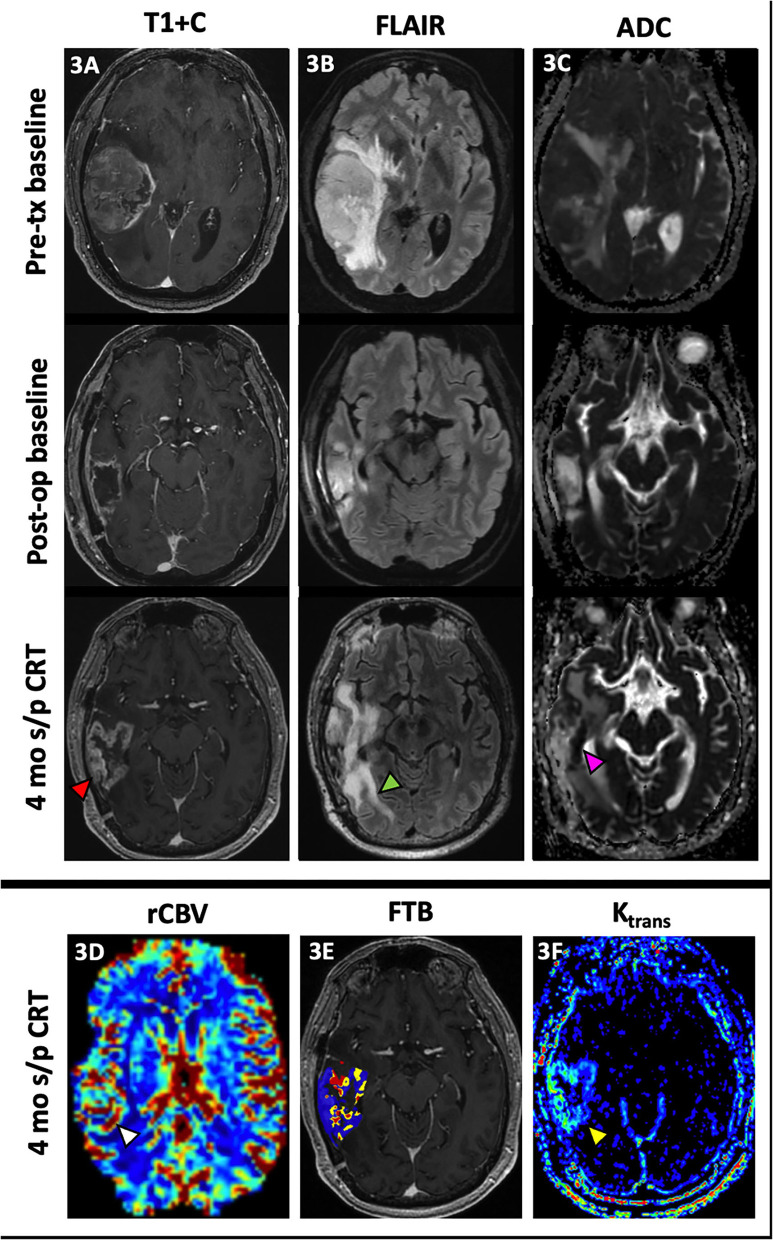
Admixture of treatment effect and tumor. A 68 year-old male with GBM (IDH wt, MGMT+) initially presented with a large enhancing right temporal lobe mass [(A), top row] with extensive perilesional FLAIR hyperintensity [(B), top row] and diffusion restriction [(C), top row]. After resection, there is mild peripheral enhancement of the resection cavity [(A), middle row] with decreased perilesional FLAIR hyperintensity [(B), middle row] and no significant diffusion restriction [(C), middle row]. At 4 mo s/p CRT, new peripheral nodular enhancement of the resection cavity [(A), bottom row, red arrowhead] is noted with interval increase in perilesional FLAIR hyperintensity [(B), bottom row, green arrowhead] and increased diffusion restriction most pronounced at the medial aspect of the resection cavity [(C), bottom row, pink arrowhead]. There is also associated increased rCBV [(D), white arrowhead], a mix of low and high fractional tumor burden (blue and red, respectively) (E) and vascular permeability [(F), yellow arrowhead]. This lesion was resected with pathology demonstrating predominantly radiation necrosis (Ki-67 of 5%) but with some neoplastic cells. The heterogeneous nature of this treated lesion is supported by the mix of low and high fractional tumor burden seen on the FTB map. ADC, apparent diffusion coefficient; CRT, chemoradiotherapy; FTB, fractional tumor burden; GBM, glioblastoma; IDH, isocitrate dehydrogenase; K^trans^, volume transfer coefficient; MGMT, O-6-methylguanine-DNA methyltransferase; mo, months; op, operative; rCBV, relative cerebral blood volume; s/p, status post; T1+C, post-contrast T1-weighted images; tx, treatment.
